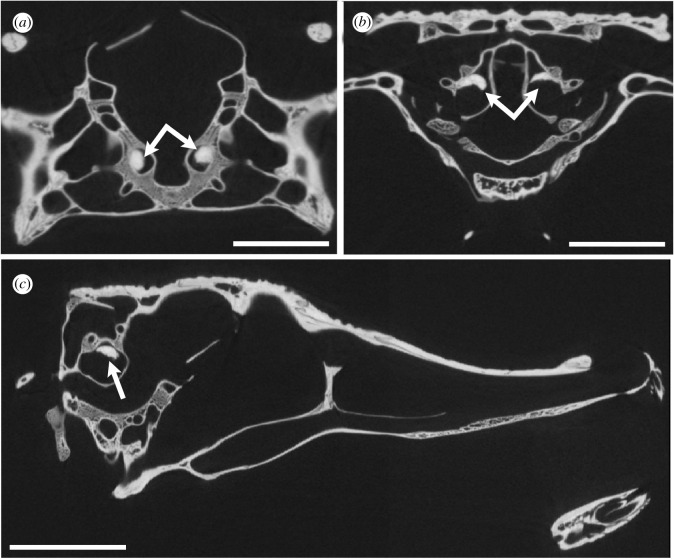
CT slices of *Osteolaemus tetraspis* (FMNH 98936) with the otolith shown in the vestibule of the bony labyrinth (white arrow). (*a*) axial, (*b*) coronal, (*c*), sagittal views. Scale bars equal 10 mm.
